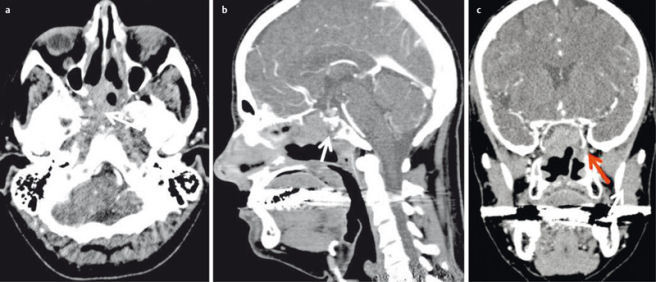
Contrast-enhanced CT of the midface of a 47-year-old patient after resection of a squamous cell carcinoma of the nasal cavity and new onset of epistaxis. a) Axial plane; recurrent disease with posterolateral infiltration of the sphenoid sinus and extension into the carotid canal (arrow). b) Sagittal plane demonstrating osseous infiltration of the clivus (arrow). c) Coronal plane with extension of the tumor into the pterygopalatine fossa (arrow).
